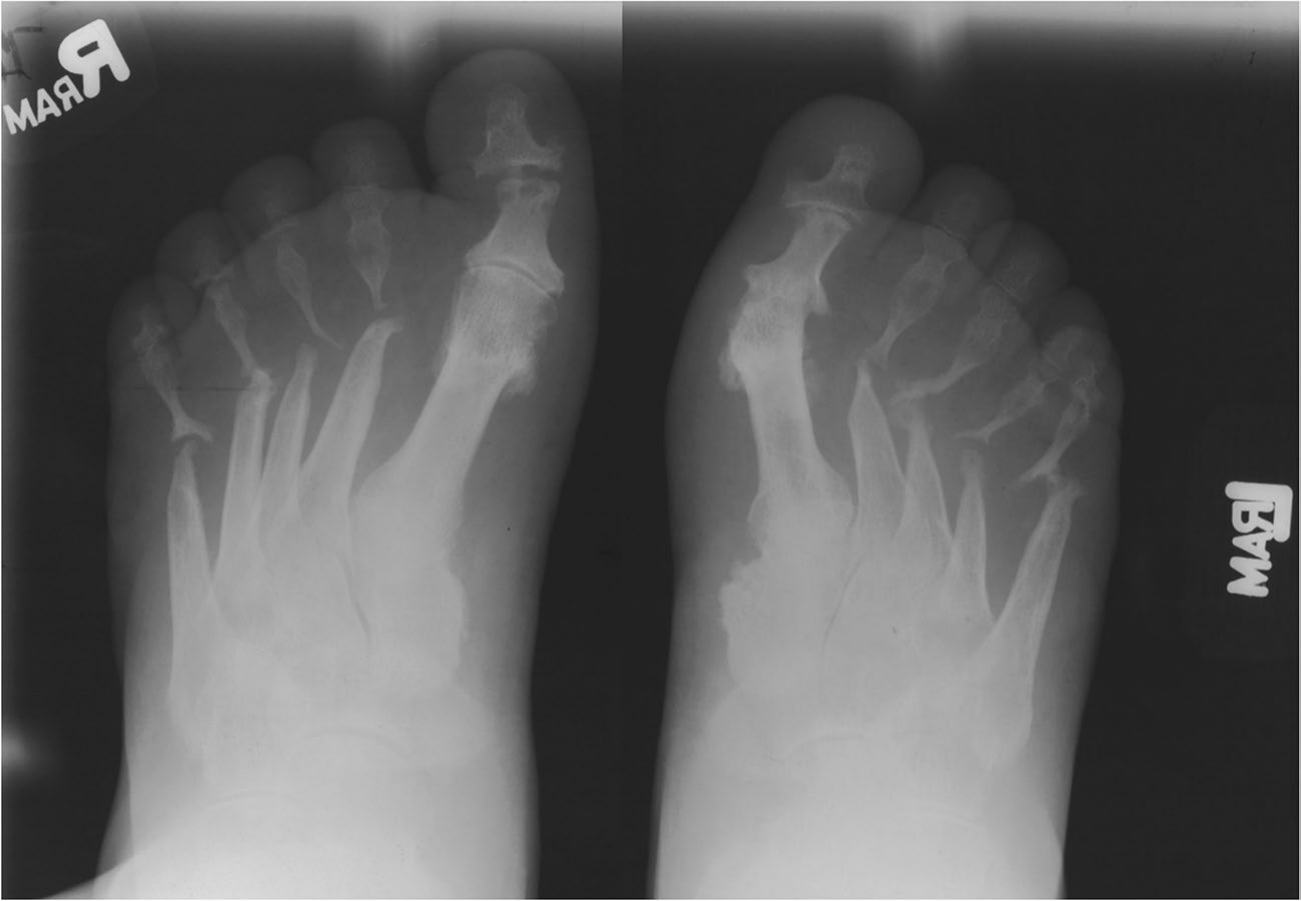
AP radiograph of the bilateral feet in a patient with psoriatic arthritis demonstrates arthritis mutilans with destructive pencil-in-cup deformities of the bilateral second through fifth metatarsophalangeal joints and fusion of the left first metatarsophalangeal and bilateral second through fifth proximal interphalangeal joints
